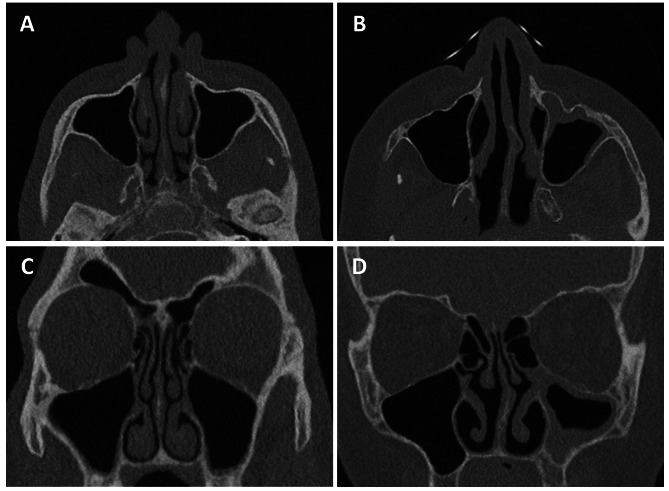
Example CT-images of the paranasal sinus for diagnosing chronic sinusitis. Shown are CT-images in axial and coronal plane in bone window of the DS 192 / 3rd Gen. + ATCM (A, C) and of the DS 192 / 3rd Gen. 2022 (B, D). Note the increased image noise and the reduced image quality with poorer delineation of bony structures in A and C
